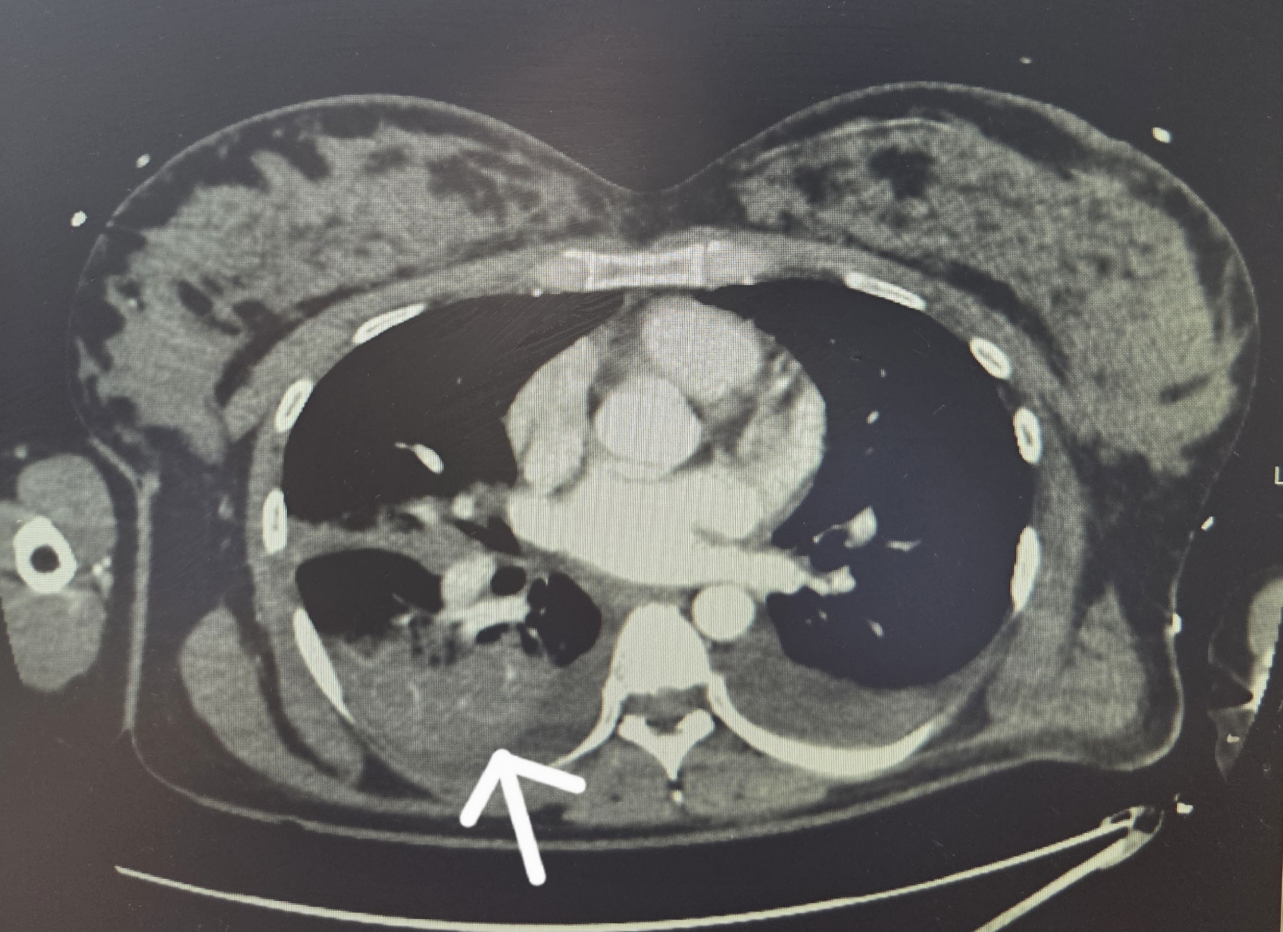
CT thorax with contrast showing dense consolidation within the right lower lobe (arrow)
